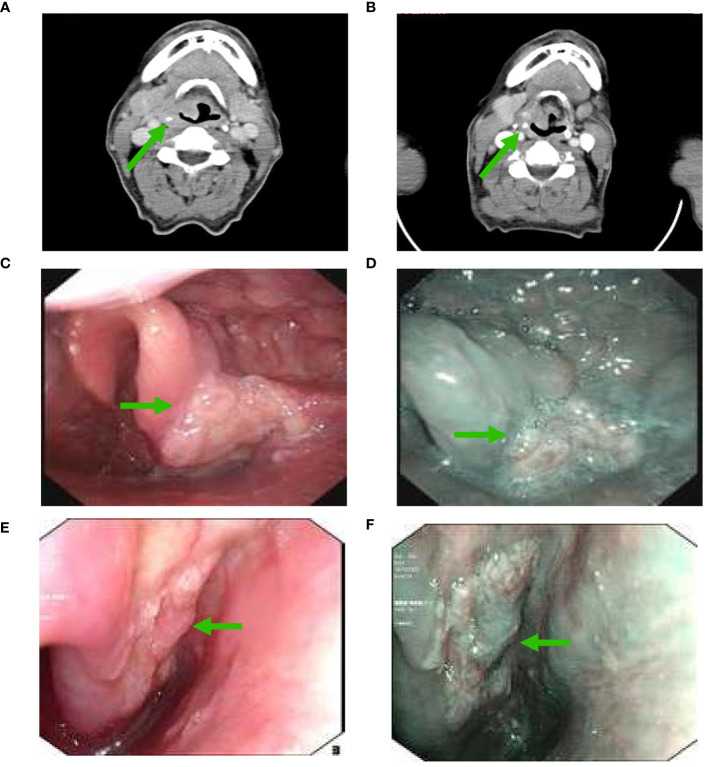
Changes in a patient with non-significant downgrading on NBI assessment after NAC and ICI (A), CT scan before NAC and ICI; (B), partial response using CT assessment after NAC and ICI; (C), white light imaging assessment before NAC and ICI; (D), NBI assessment before NAC and ICI; (E), white light imaging assessment after NAC and ICI; (F), a non-significant downgrading on NBI assessment after NAC and ICI (CT, computed tomography; NAC, neoadjuvant chemotherapy; ICI, immune checkpoint inhibitor; NBI, narrow band imaging) (The green arrow refers to the primary hypopharyngeal lesion).
